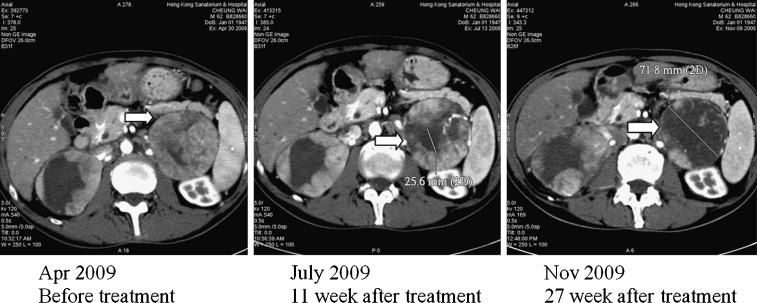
Computerized Tomographic (CT) Scan of HCC Patient: Serial CT scan images of liver tumor before treatment, 11 weeks after treatment showing the increase of necrosis with diameter of 25.6 mm, and 27 weeks after treatment showing necrosis with diameter of 71.8 mm, respectively
